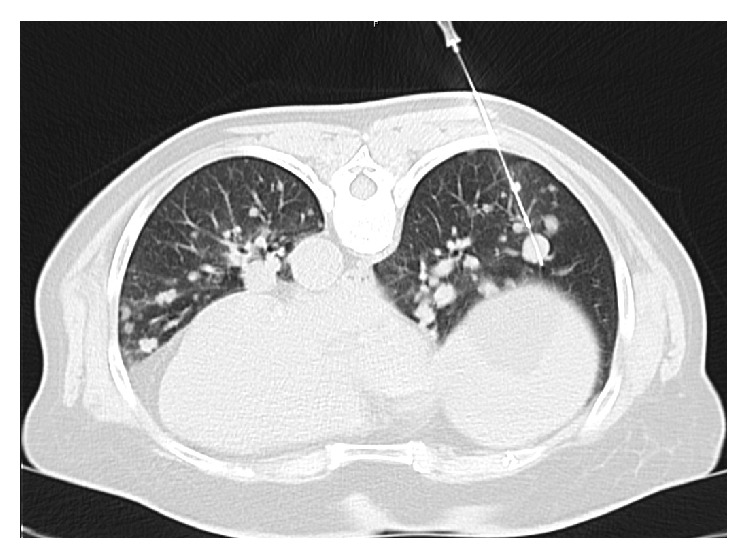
CT-guided needle biopsy of pulmonary metastasis of splenic angiosarcoma (20 G Trucut needle).
